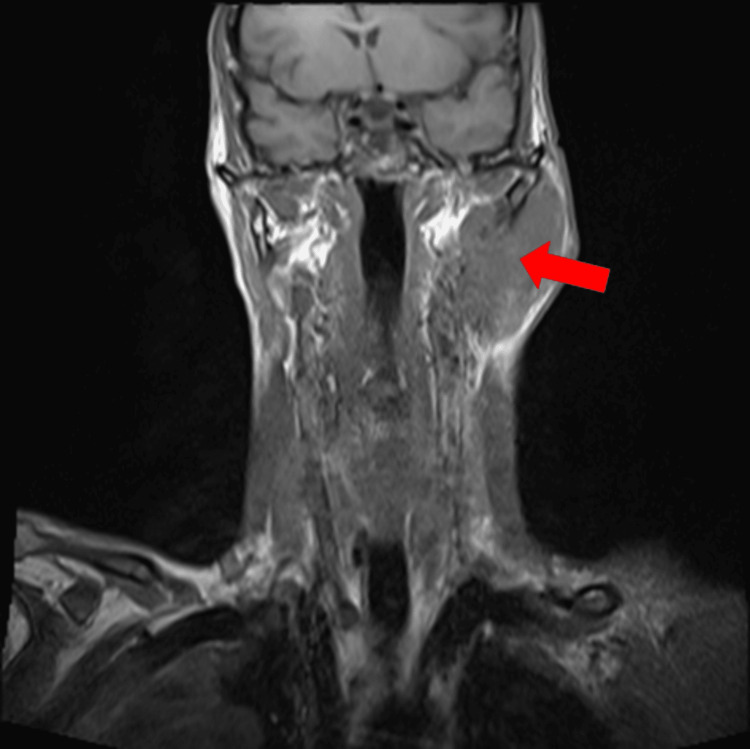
Head and neck MRI. A voluminous formation, apparently solid and vascularized, with an infiltrative and relatively non-destructive character, with great expansion of the parotid gland, the neck muscles, especially the sternocleidomastoid.
